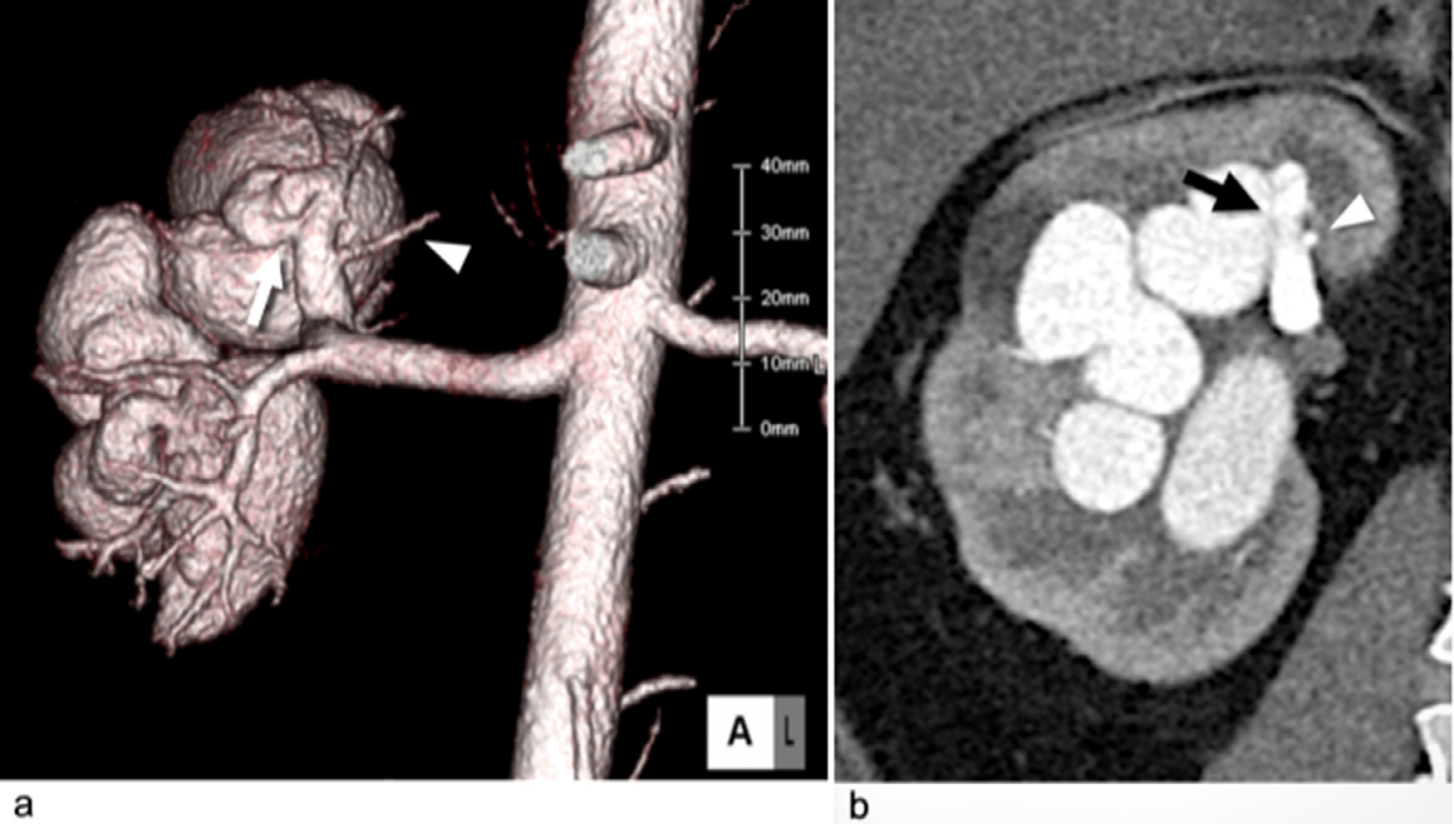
A 25-year-old male patient with right renal arteriovenous fistulas (r-AVF) in Case 1. (A) Volume rendering of computed tomography (CT) angiography showing a huge right r-AVF with a dilated venous sac near the fistula (white arrow) and early venous return. The white arrowhead indicates a normal branch proximal to the fistula. (B) An arterial-phase coronal CT image showing a huge right r-AVF with a venous sac connecting the dilated renal vein. The fistula (black arrow) is proximal to the normal branch (white arrowhead).
